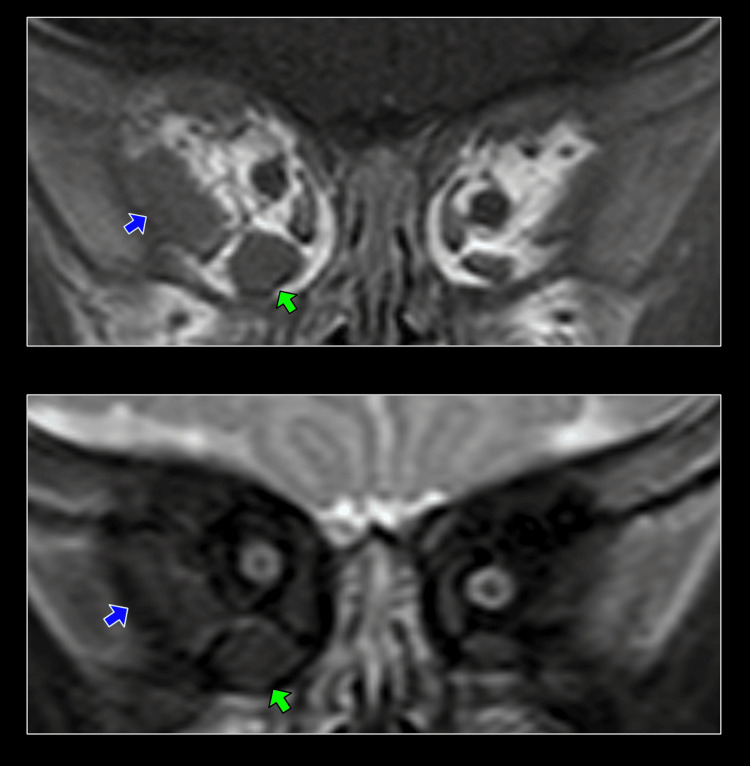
MRI and Short Tau Inversion Recovery (STIR) image at the first visit. The T1 phase of the MRI orbit revealed enlargement of the lateral rectus and inferior rectus. (Upper images) The blue arrow is the right lateral rectus muscle, and the green arrow is the right inferior rectus muscle. There are no inflammatory findings on the lower images (STIR images). The blue arrow is the right lateral rectus muscle, and the green arrow is the right inferior rectus muscle. MRI, magnetic resonance imaging
